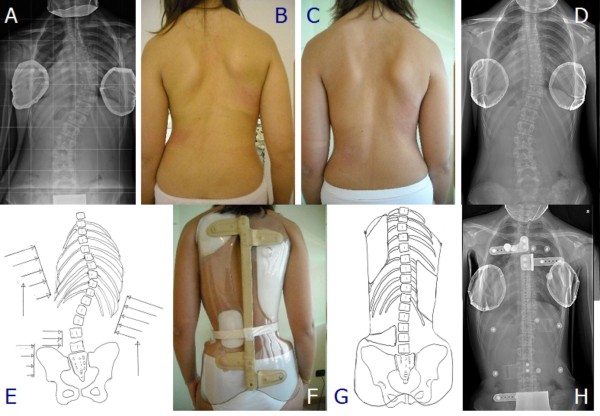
Example of correction on the frontal plane slopes. Example of correction on the frontal plane slopes of one patient with a thoracic curve of 48° (Risser 1). (A) X-ray pre-brace (48°); (B) aesthetics pre-brace; (C) aesthetics after 4 months of bracing; (D) x-ray without the brace after 4 months of bracing: corrected to 23° (i.e. reduction of 25°, -52%); (E) pre-brace planning with pushes on the right thoraco-lumbar and left thoracic slopes, as well as a stop to the right of the flanks; (F) constructed brace; (G) application of the pushes in the constructed brace; (H) in-brace x-ray with a correction to 13° (i.e. 35° of correction, 73%).
